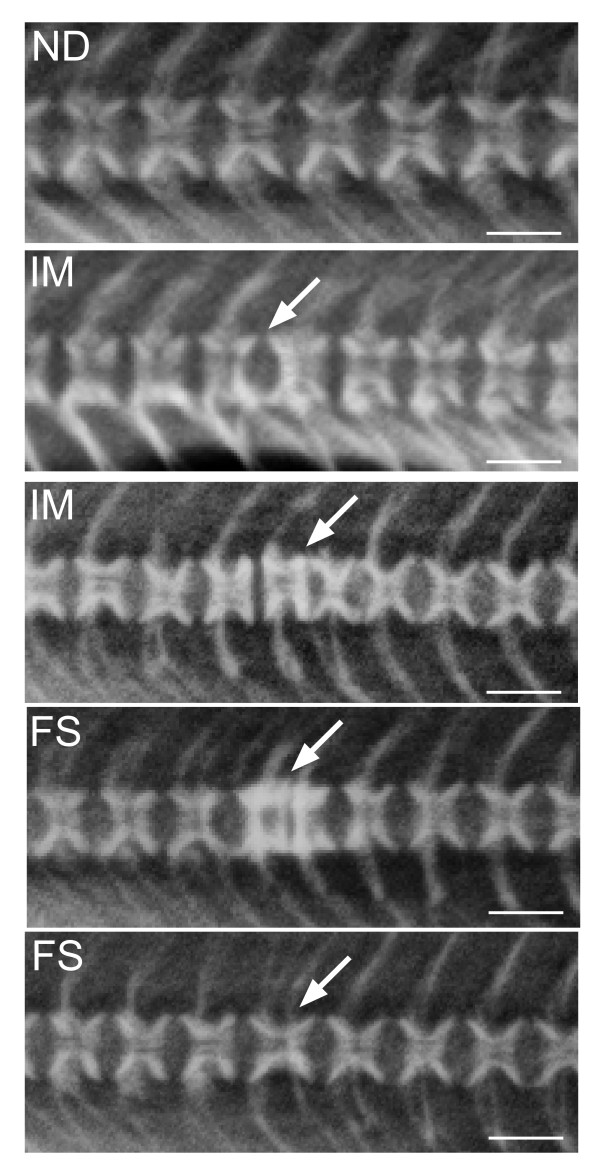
X-ray and diagnostics. The development of vertebral fusions is a dynamic process where the final result is a complete fusion of two or more vertebral bodies. Vertebrae were divided into non-deformed (ND), intermediate (IM) and fused (FS). White arrow points to malformed vertebral bodies. Scale bar = 0.1 cm.
